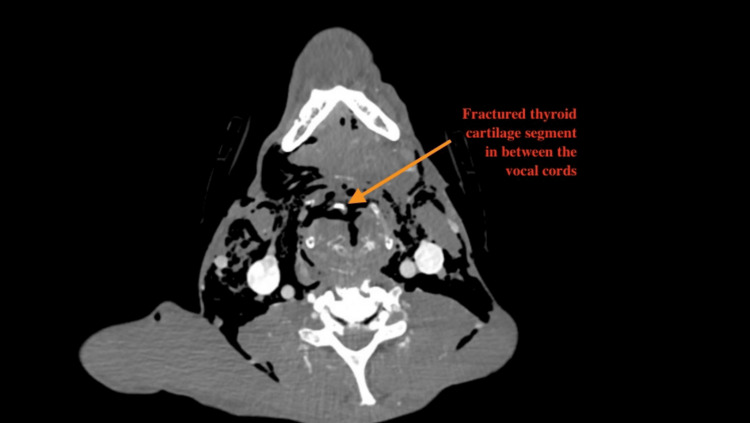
Pre-operative neck CT scan (axial view) The arrow indicates the fractured thyroid cartilage segment separated from the thyroid cartilage.
